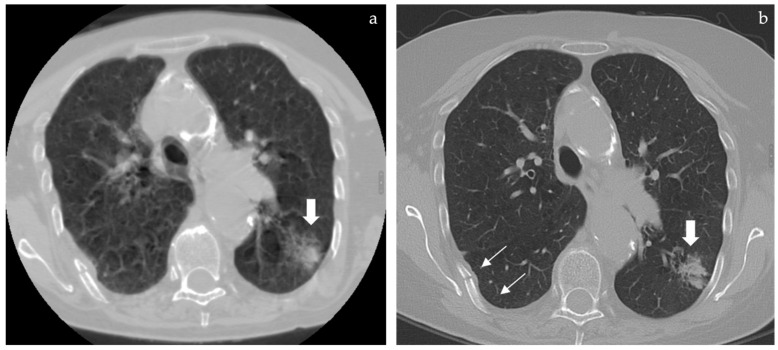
(a) (left) and (b) (right): whenever a lung opacity is spotted by chance in spine CT a full FOV reconstruction can be performed but only if RAW data are still available in the scanner. In the case (previously described in Figure 1) this allowed us to rule out further opacities but the respiratory motion artifacts prevented a proper characterization of the main lung finding that appeared as a blurry edged opacity (a, large arrow). The patient was called back and a standard thoracic CT was repeated to better define the lung finding that appeared as a subsolid ground glass opacity compatible with a lepidic growth adenocarcinoma (b, large arrow) as later confirmed by lung biopsy. In both full FOV reconstructions a rib fracture was detected (b, thin arrows).
